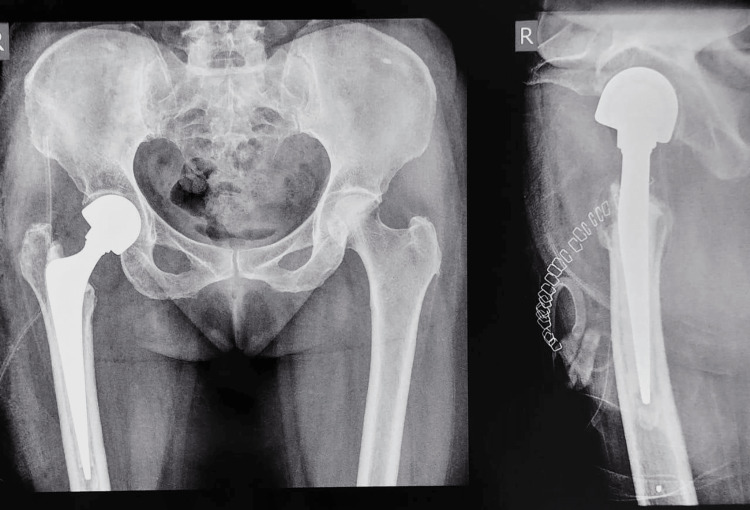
Postoperative X-ray - Right-sided cemented bipolar hemiarthroplasty The X-ray shows the patient is operated on with a right-sided bipolar hemiarthroplasty; the radiolucent shadow of metastatic lesion in the preoperative X-ray is absent and has been replaced with the bipolar hemiarthroplasty prosthesis; the prosthesis has an intramedullary radioopaque shadow surrounding the stem of the bone cement; and in the lateral X-ray, a radioopaque dot can be seen of the cement restricter.
